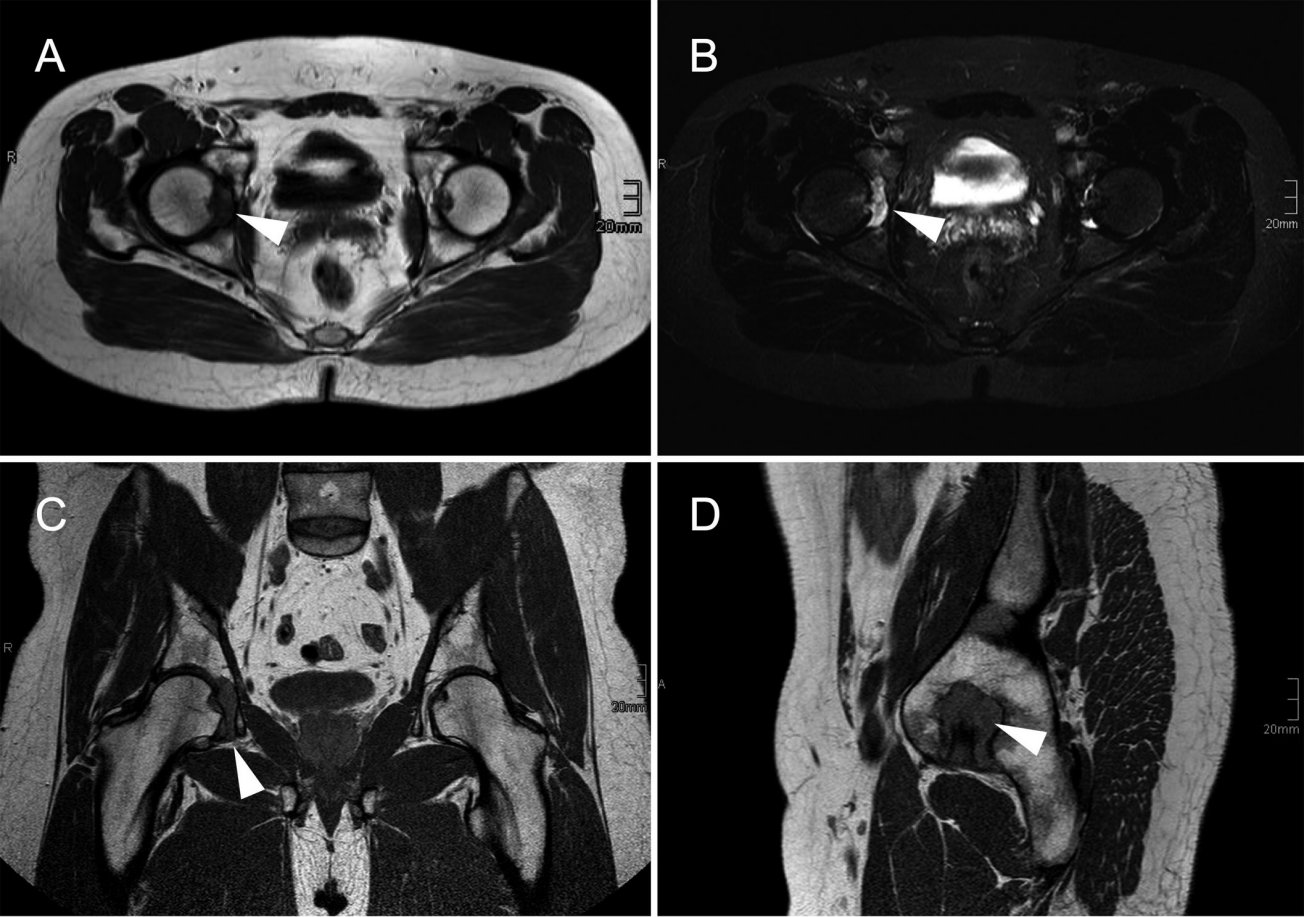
Un-enhanced MR images acquired with a T1-weighted TSE sequence in transversal (A), coronal (C), and sagittal (D) orientation. The tissue within the joint space (arrows) appeared hyperintense, comparable to skeletal muscle, and seemed to arise from the caudal parts of the joint's capsule. The ligamentum capitis femoris was well differentiated from the mass. In the fat-suppressed T2-weighted TSE images (B), inhomogenous hyperintensity of the mass was apparent. Remarkably, there was no signal loss within the mass indicating areas of calcification.
